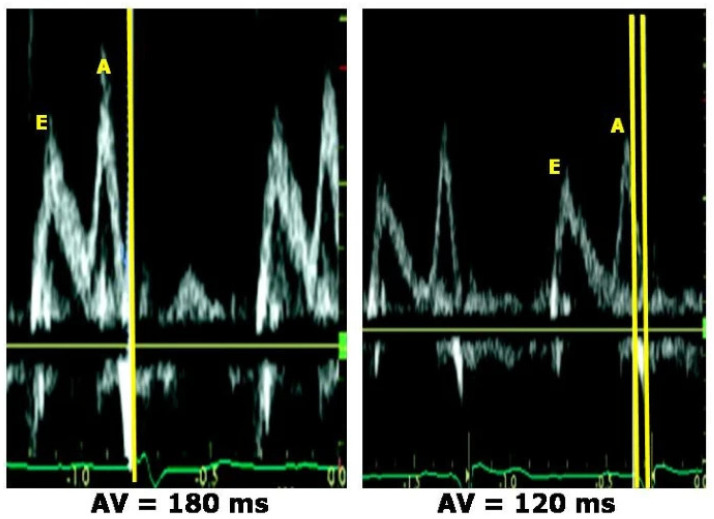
Optimization of AV delay after CRT by pulsed Doppler mitral inflow pattern. (Photo extracted from Galderisi, M. et al. Doppler echocardiography and myocardial dyssynchrony: a practical update of old and new ultrasound technologies. Cardiovasc Ultrasound 5, 28 (2007)) [25].
